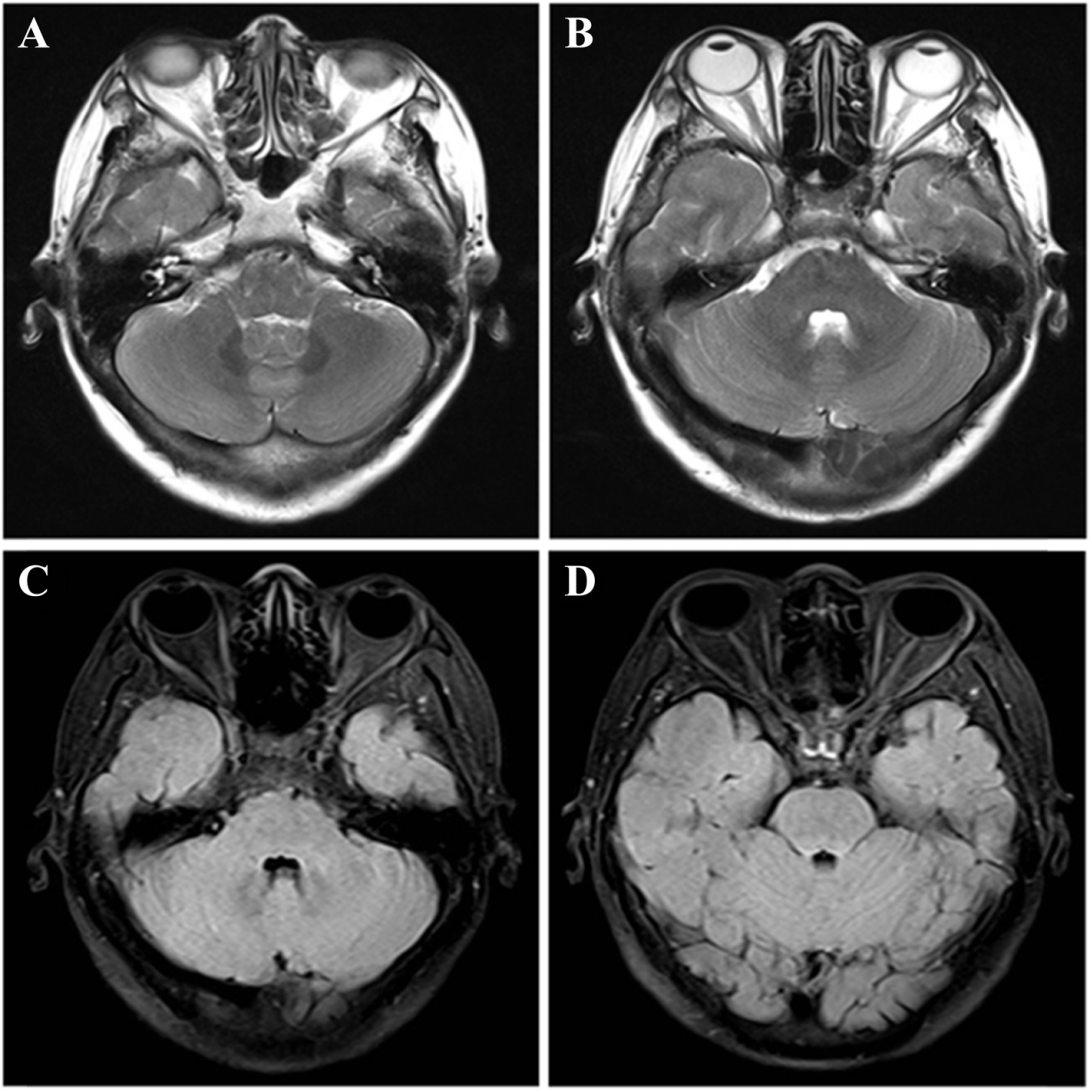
the patient’s MRI of the brain showed no damage of extraocular muscle. (A, B) T2WI axial image. (C, D) T1WI axial image
